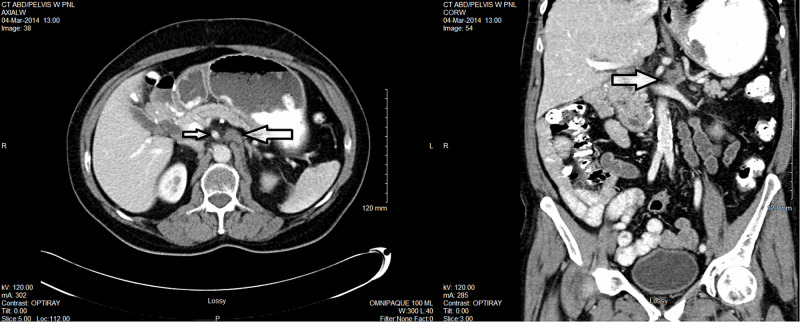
CT scan in March 2014 showing good PR. Note the residual mass (big arrow) and SMA (small arrow). PR, partial response; SMA, superior mesentery artery
